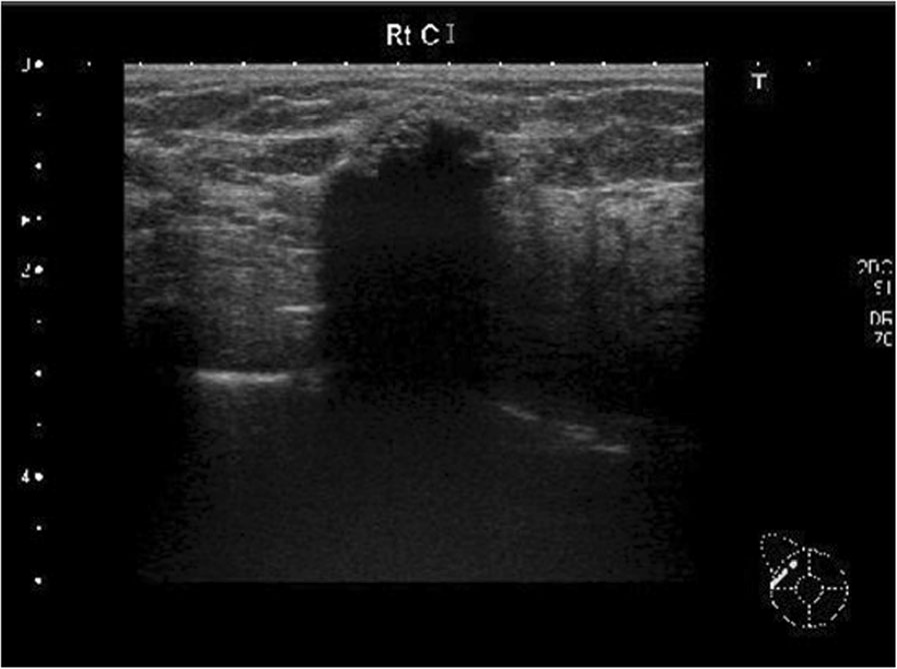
Ultrasonography. A 1.7-cm-diameter relatively smoothly marginated hypoechoic mass containing multiple calcifications
